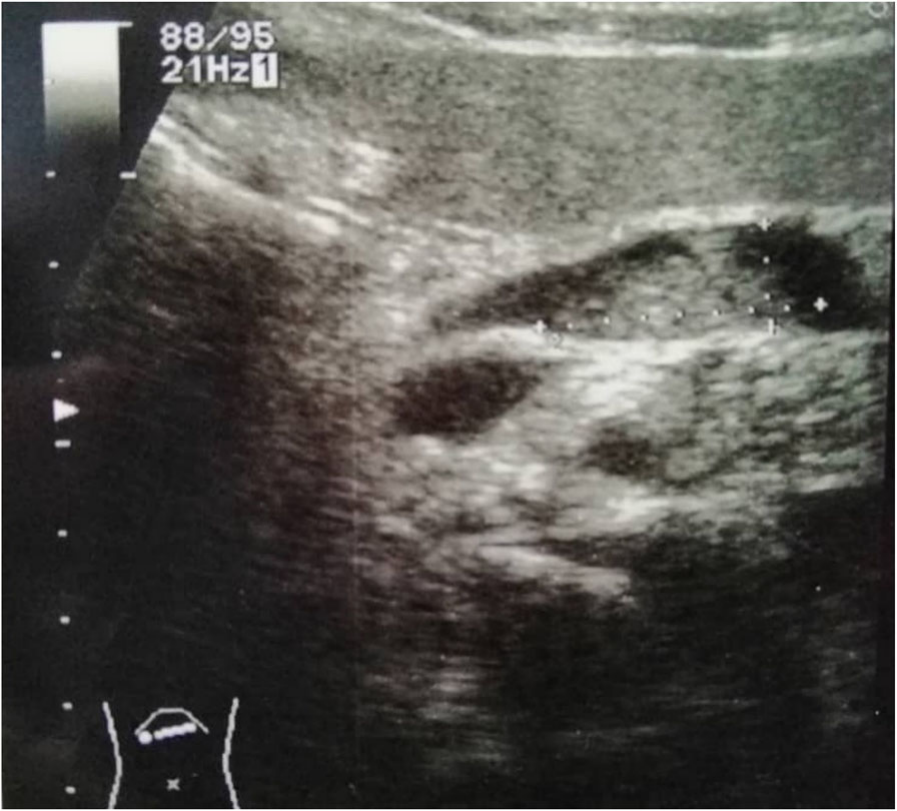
Abdominal ultrasound revealing a cystic dilation in the main pancreatic duct
